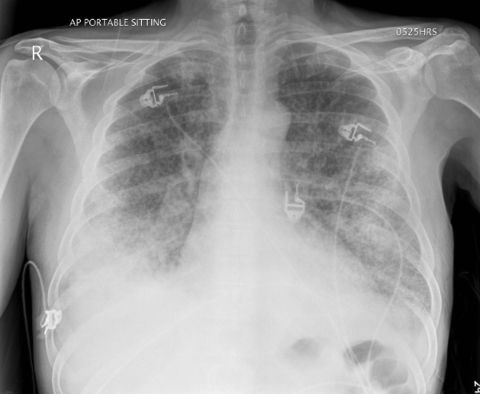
Chest radiograph of patient 11 at time of admission to hospital, before intubation, demonstrating extensive bilateral airspace disease.
